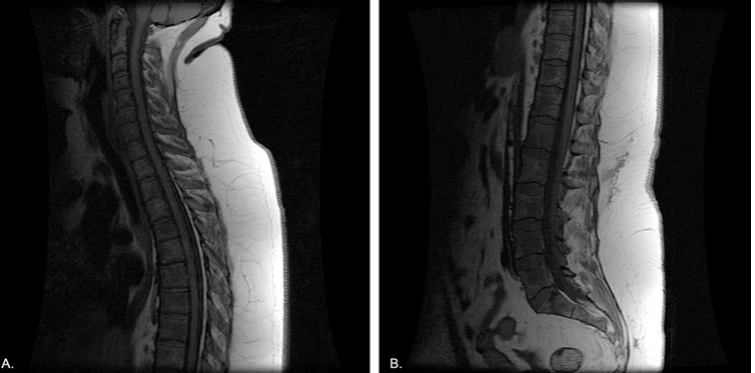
MRI showing (A) cervico-thoracic involvement and (B) thoraco-lumbar involvement. T2 weighted image highlighting heterogenous involvement of the entire spine with preserved disk space and absence of fractures.
